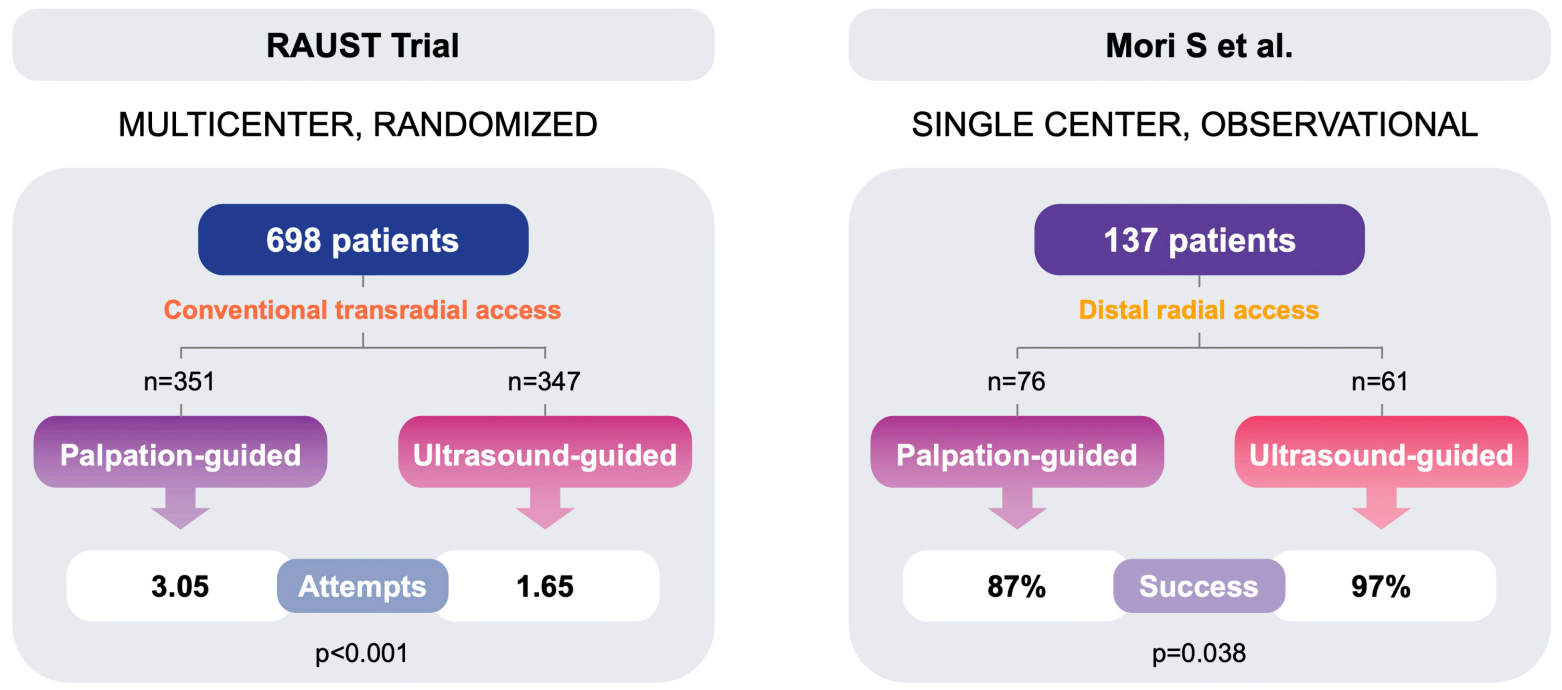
Results of ultrasound-guided radial access. The left panel
